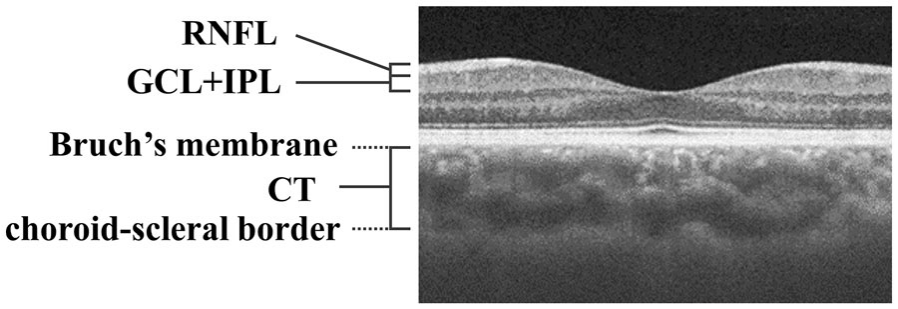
Enlarged image of the macula from Fig. 1. The layer above the retina, characterized by high brightness, is the retinal nerve fiber layer (RNFL), while the underlying layer is the ganglion cell layer + inner plexiform layer (GCL + IPL). Choroidal thickness (CT) was defined as the distance from Bruch’s membrane to the choroid-scleral border
